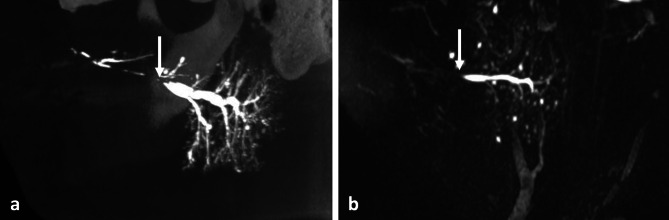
Imaging of the left parotid gland showing a stenosis (white arrow) of the pre-masseteric portion of the Stensen’s duct visible in MIP sagittal view with 3D-CBCT sialography (a) and MR sialography (b), upstream ductal dilatation is observed in sialography, as for intraglandular multiple cystic dilatations in both imaging modalities
